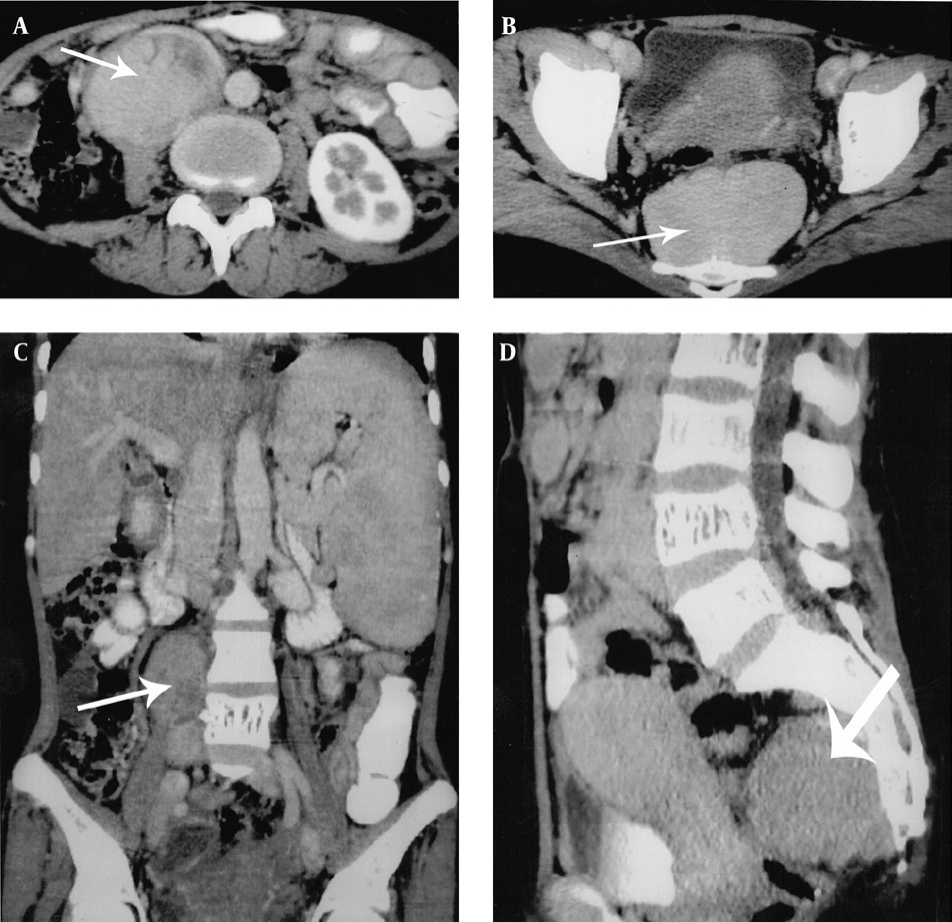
Abdominopelvic MDCT; axial sections show intensely enhancing soft tissue lesions in the right paraspinal region (A) and the presacral region (B). Coronal reformatted CT image (C) shows moderate splenomegaly and intensely enhancing soft tissue lesion in the right paraspinal region. Sagittal reformatted CT image (D) shows intensely enhancing soft tissue lesion in the presacral region.
